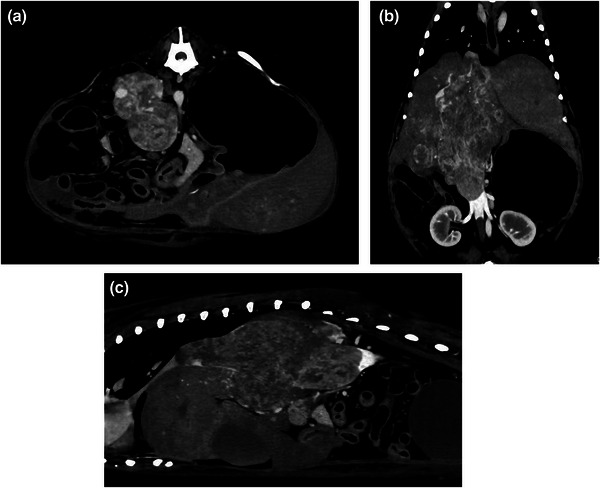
Preoperative computed tomography images. (a) Cross‐sectional image. (b) Coronal image. (c) Sagittal image. The tumour arising from the right adrenal gland not only invades the surrounding tissue but also forms a large caval thrombus in the caudal vena cava, spreading into the right hepatic vein and accessory right hepatic vein. The tip of the caval thrombus reaches the diaphragm; moreover, there is poor perfusion of the left and central hepatic veins and reduced hepatic blood perfusion, which resulted in a poor contrast‐enhancement.
